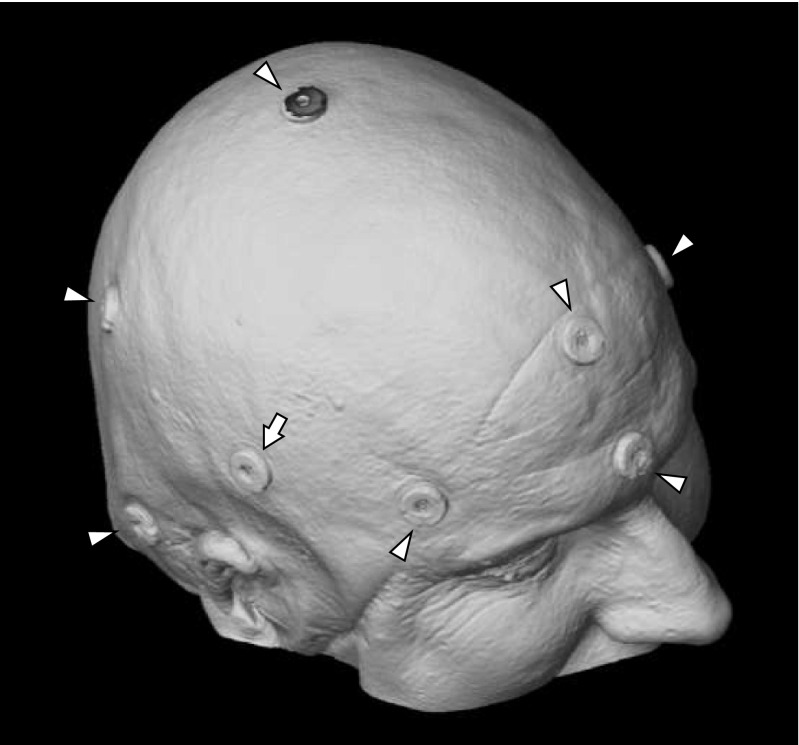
Three-dimensional MRI reconstruction demonstrating a typical distribution of adhesive markers. The seven markers for registration are indicated by arrowheads. The arrow depicts the additional “target-marker” where surgical entry is to be expected (close to the pathology). In this case, the Curve was used for navigation, and TRE values were as follows: adhesive markers, 3.30 mm; surface matching, 6.00 mm; and second surface matching attempt, 6.30 mm
